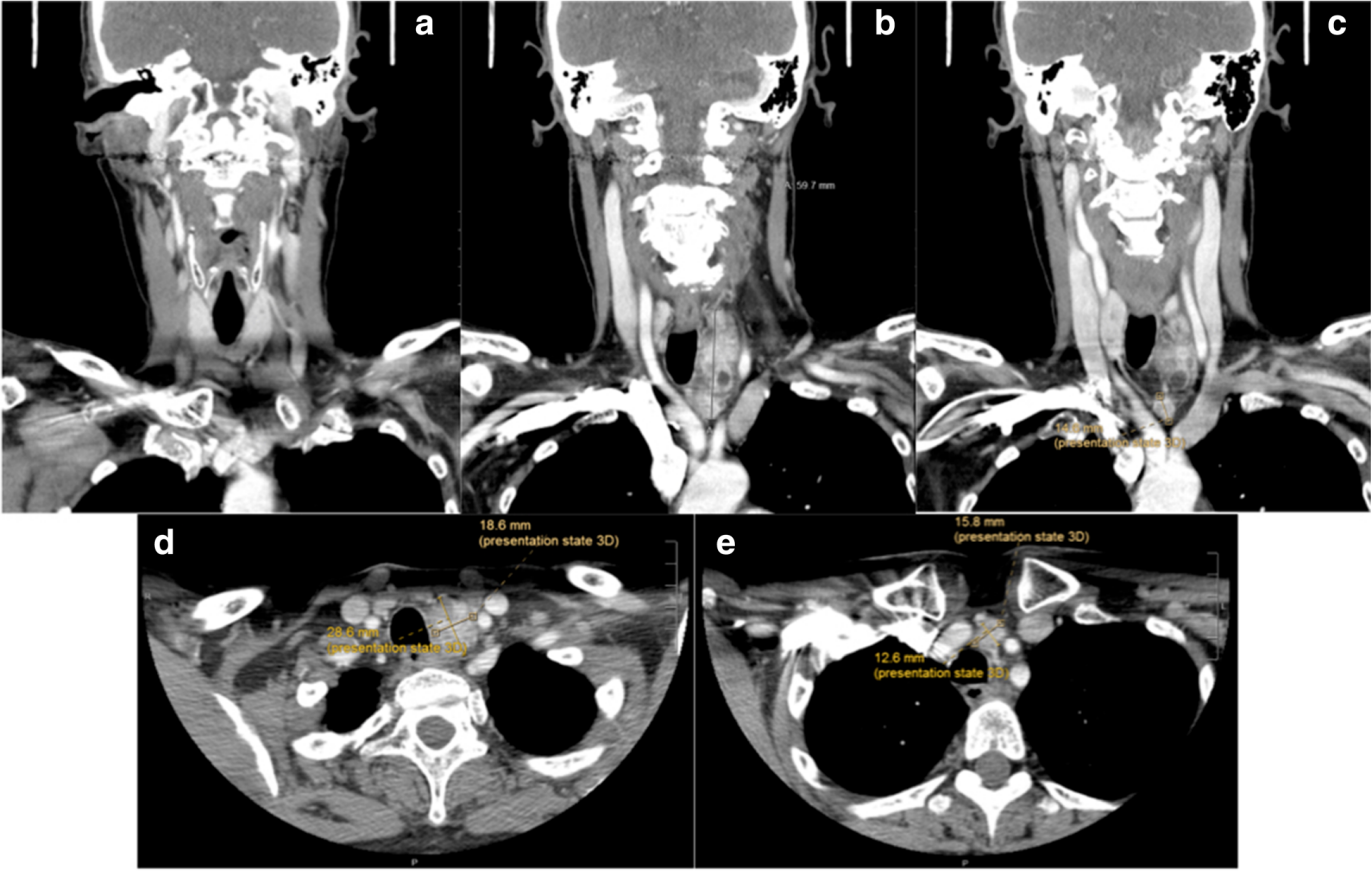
Computed Tomography scan of the neck with IV contrast showing the primary parathyroid lesion and the separate supra-aortic parathyroid lesion, likely secondary to seeding from FNA. a, b, c - Coronal view; d, e - Axial view. The normal thyroid gland is identified (a). The primary parathyroid lesion, measuring 59.7 mm, is located posterior to the thyroid gland (b). The separate supra-aortic lesion likely secondary to seeding measures to be 14.6 mm (c). The primary parathyroid lesion measures 18.6 mm x 28.6 mm (d). The separate supra-aortic lesion localized in the pre-tracheal area measures 12.6 mm x 15.8 mm (e)
